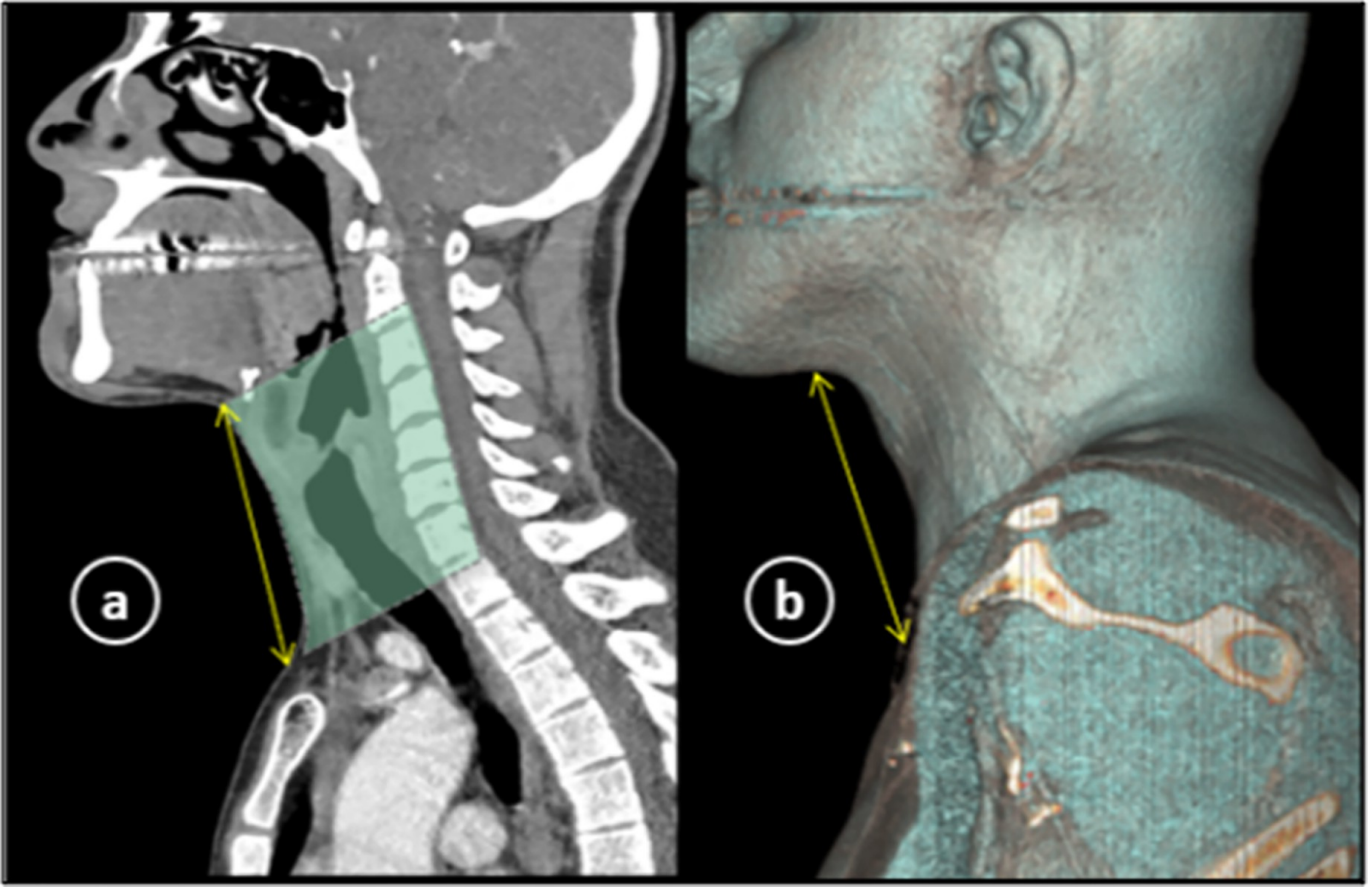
CT neck sagittal section (a) and its 3D volumetric reconstruction (b) showing how anterior neck length was measured (double arrow line).
